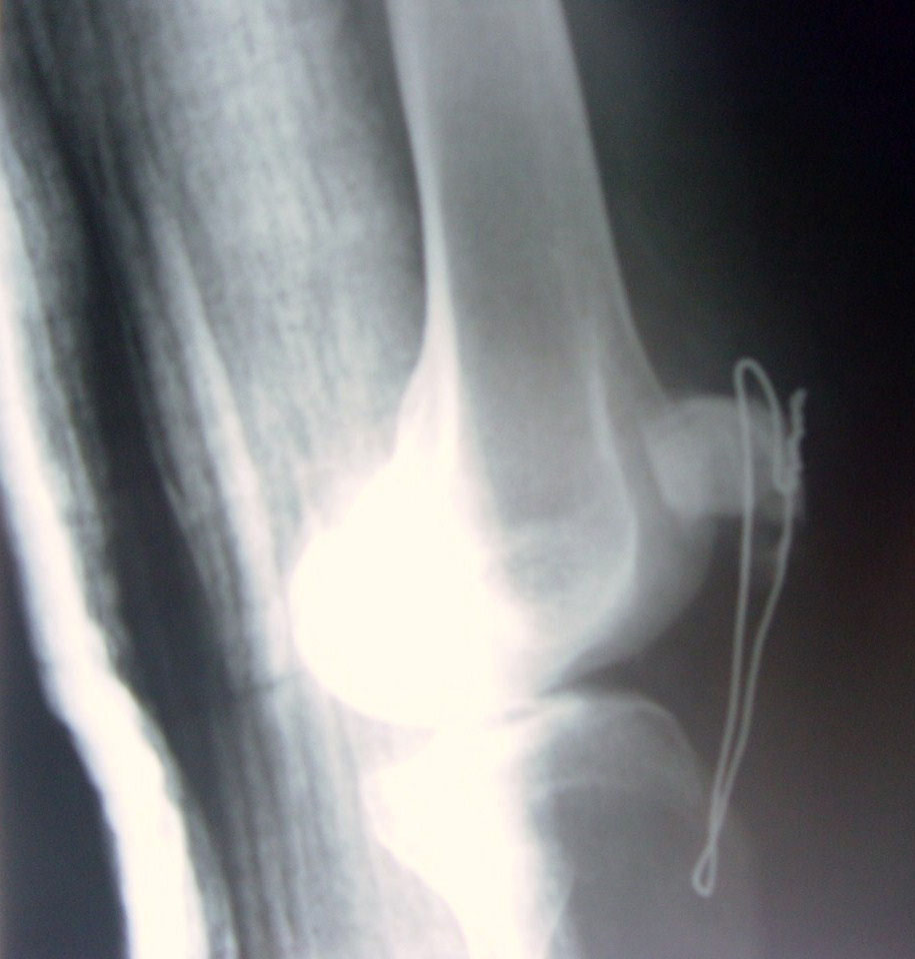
Postoperative lateral radiograph of the patient.
